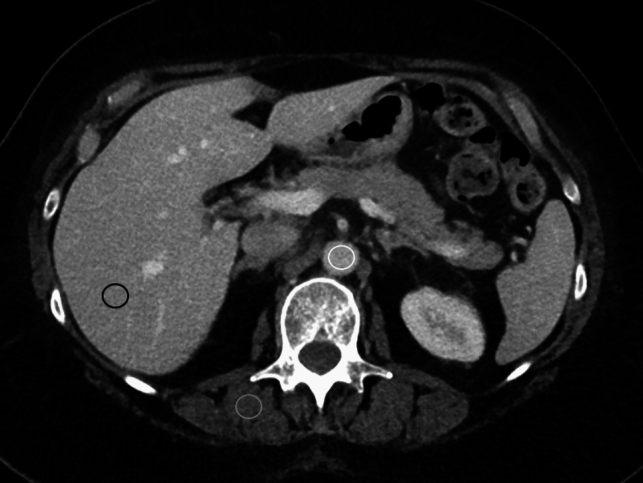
Axial CT image at the level of the first lumbar vertebra, on which regions of interest were circled within the abdominal aorta (white circle), the right liver lobe (black circle) and the paraspinal right muscles (gray circle) to evaluate objective image quality
